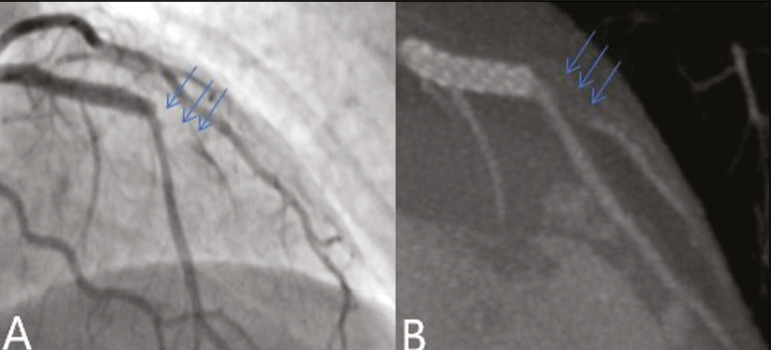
Patient with an unobstructed stent in the ADA, together with thrombus and occlusion at the origin of the diagonal branch of the ADA. In this case, the modifier S (for “stent”) is added (CAD-RADS 5S). A: Catheterization. B: CTA.
